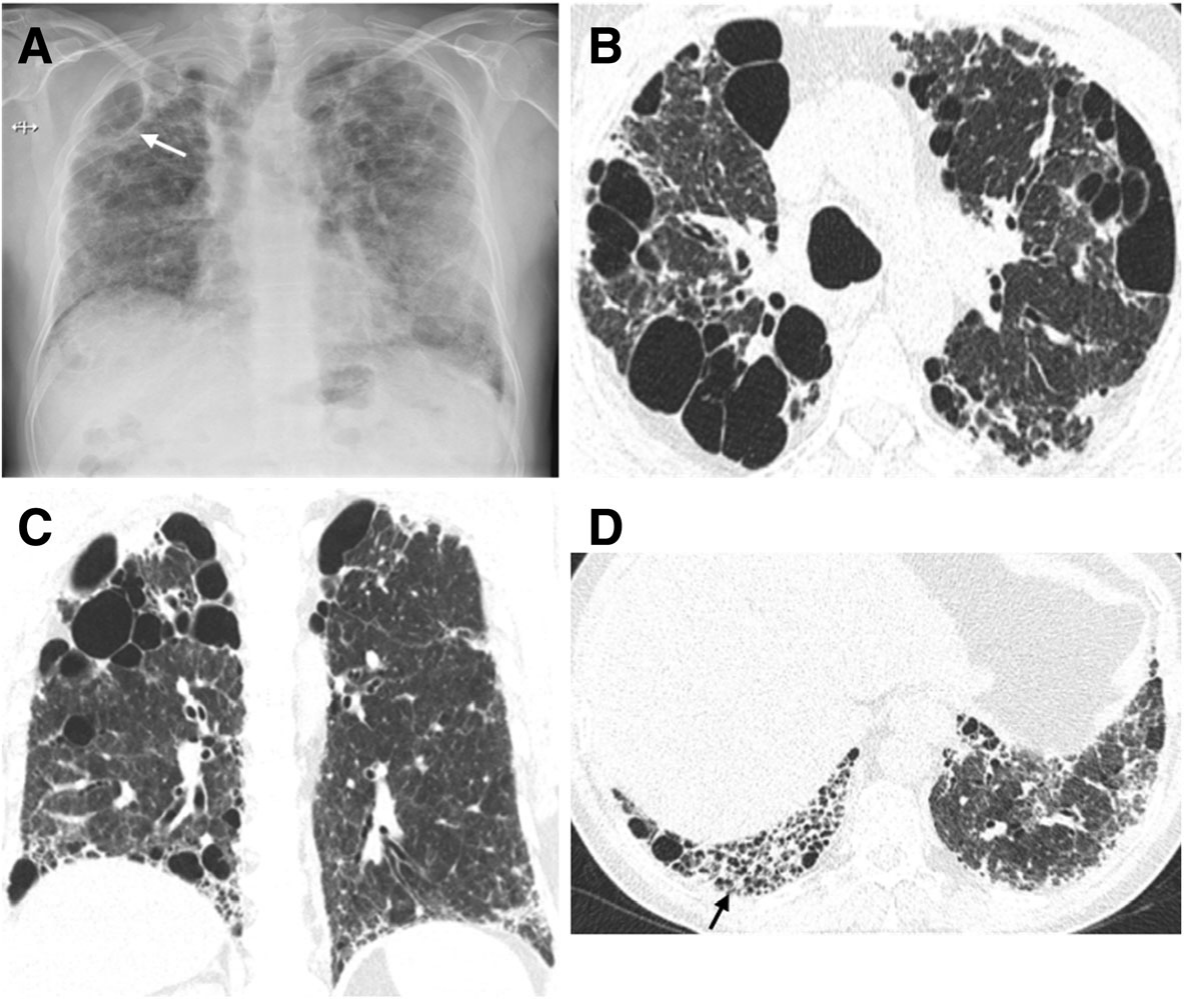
Combined pulmonary fibrosis and emphysema (CPFE). a Posteroanterior (PA) radiograph shows right volume loss, diffuse bilateral reticular opacities, and GGO. There are lucencies in the upper lobes (white arrow), signifying bullous emphysema. b Axial and c coronal CT images show centrilobular, paraseptal, and bullous emphysema predominantly in the upper lobes. d Axial image through the lower lobes shows peripheral predominant fibrosis with reticulation, traction bronchiectasis, and honeycombing (black arrow), in a UIP pattern
